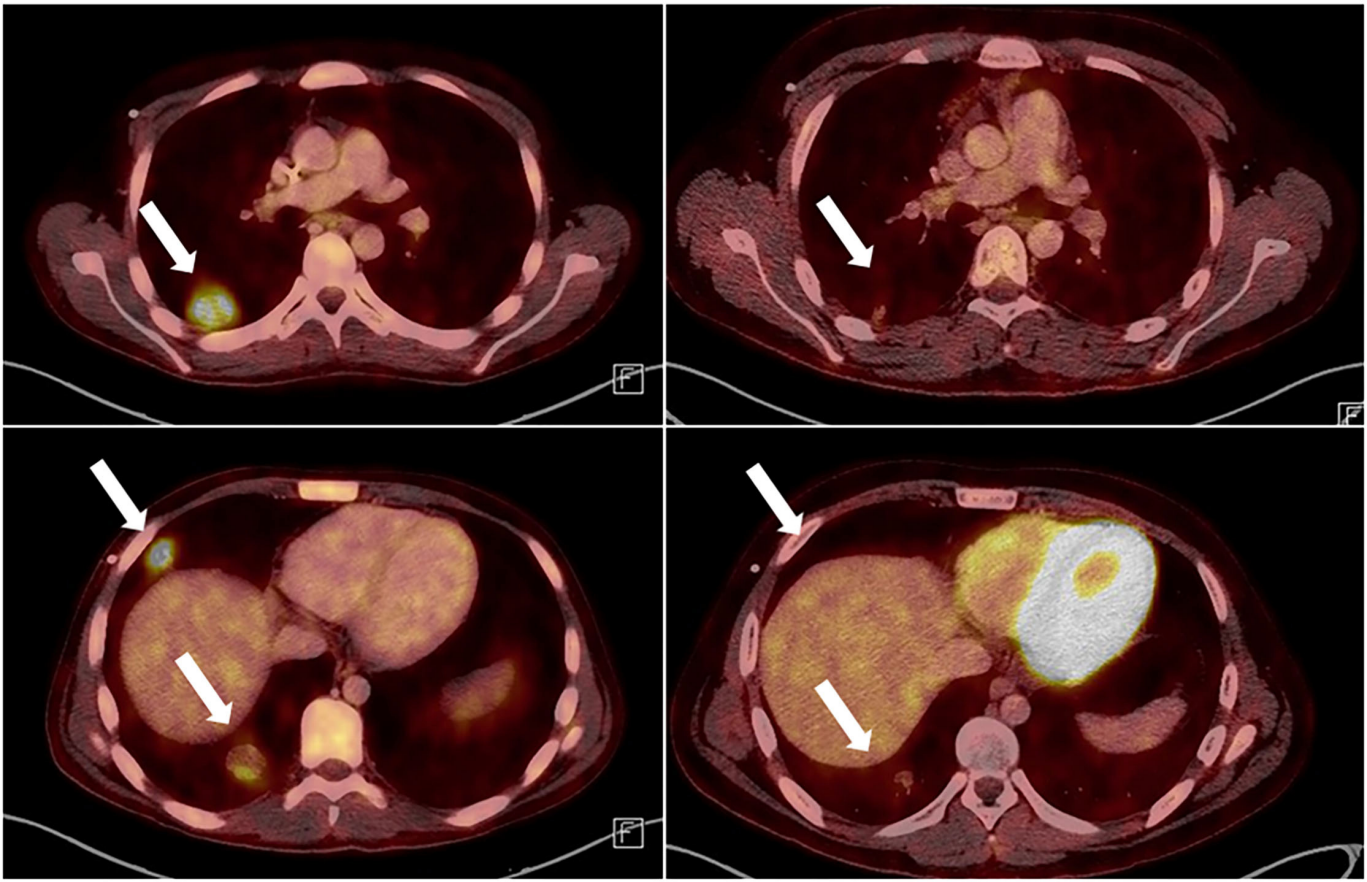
Thoracic FDG-PET CT prior to treatment (left side) and after 5 cycles or Rituximab (right side). Lesions marked with arrows. The luminous structure in the lower right picture is the left ventricle, which shows an increased metabolism compared to the right picture, because it is contracting. This FDG-PET accumulation has nothing to do with the metabolism of the tumor cells.
